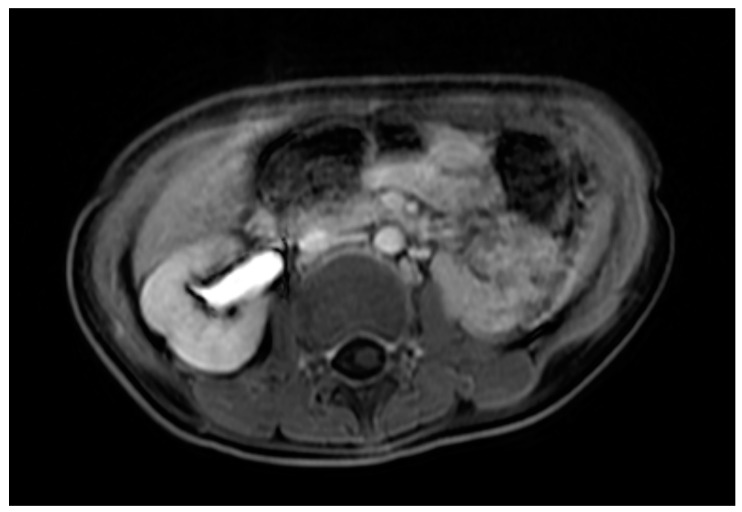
MRI of case 3: left renal agenesis and right hydroureter.
